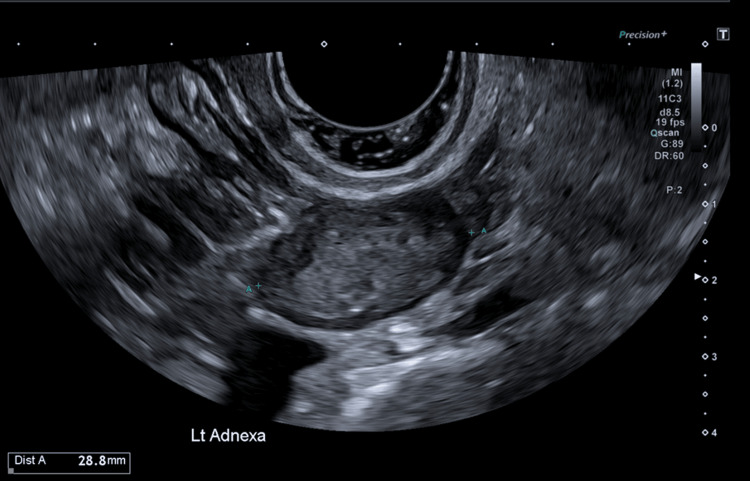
Transvaginal ultrasound demonstrating a homogeneous solid echogenic mass in the left adnexa measuring approximately 2.7×1.8×2.9 cm
